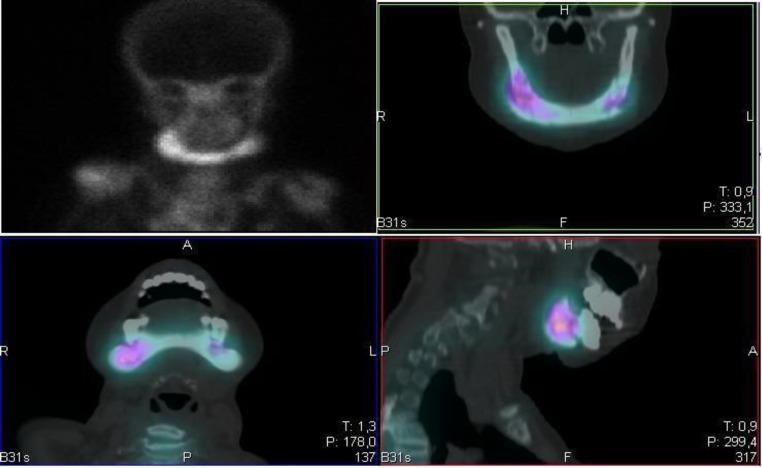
Bone scintigraphy with technetium-99m showing increased blood flow to the mandible, with diffuse heterogeneous uptake of the radiopharma- ceutical in marked degree to the fullest extent of the mandible, compatible with osteomyelitis in activity
